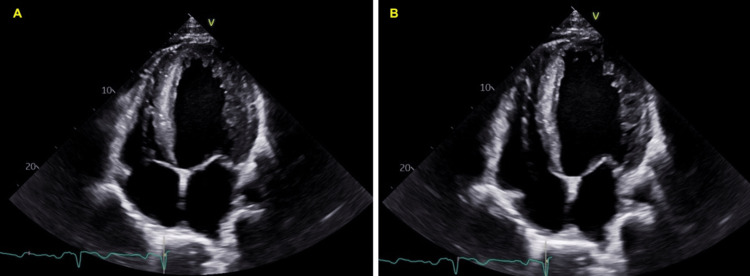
Apical four-chamber view of transthoracic echocardiogram (A) systolic phase and (B) diastolic phase with decreased left ventricular ejection fracture of 20-25%, with normal interventricular wall thickness (1 cm) and mild mitral regurgitation.
